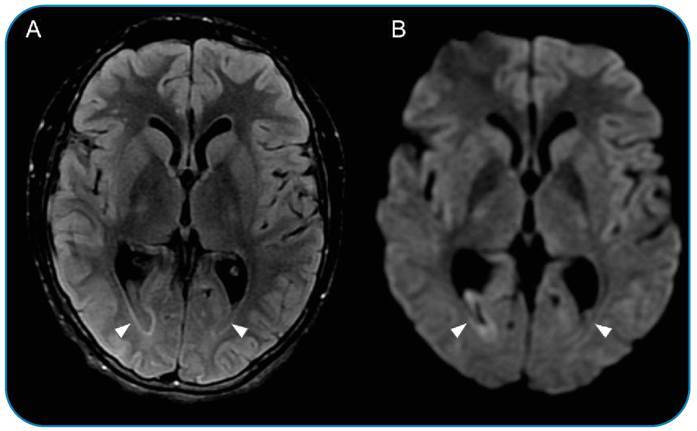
Magnetic resonance images of the brain show intermediate signal content in the lateral ventricles at FLAIR sequence (A) with restricted diffusion at DWI (B).
